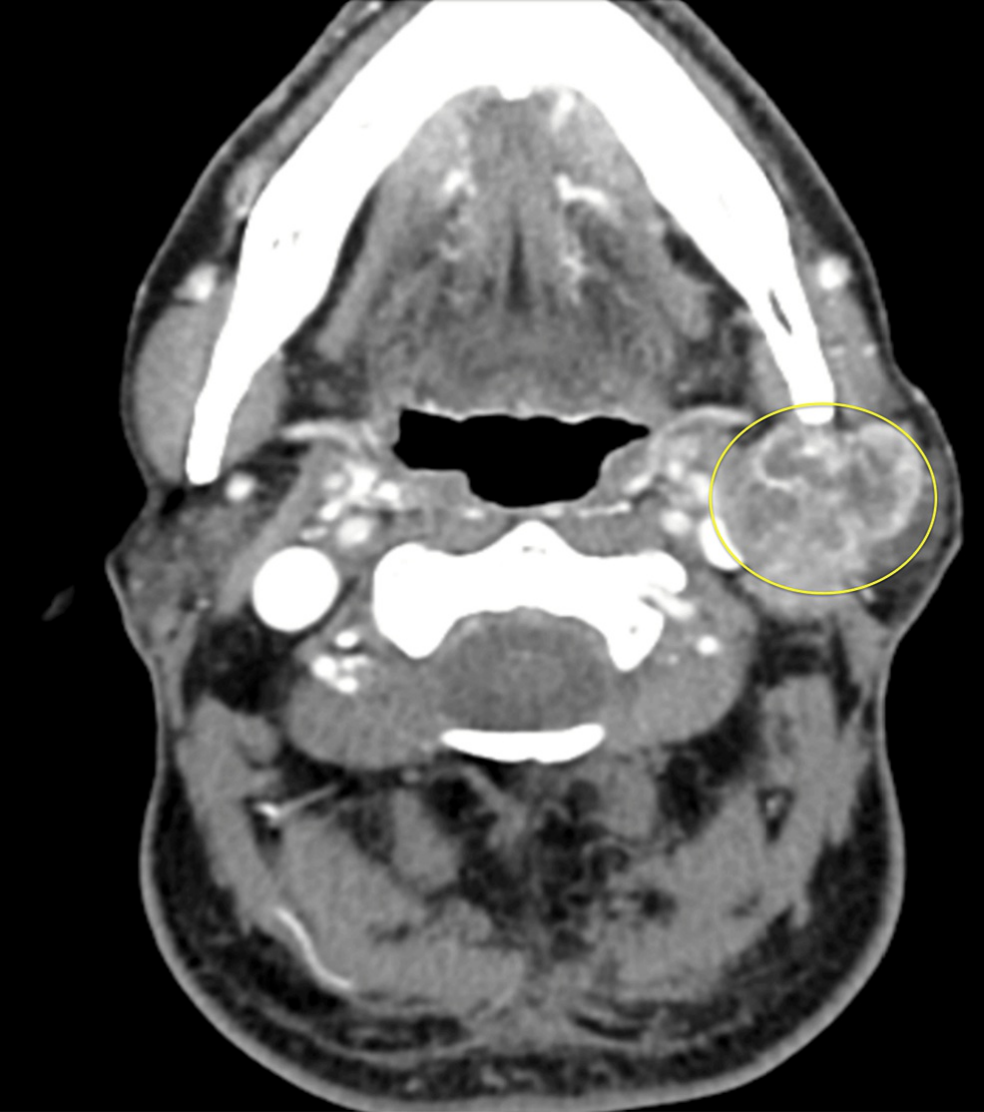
Axial-cut contrasted CT neck demonstrating a retromandibular, deep lobe parotid mass for Patient 2 Yellow circle: retromandibular, deep lobe parotid mass CT: computed tomography
